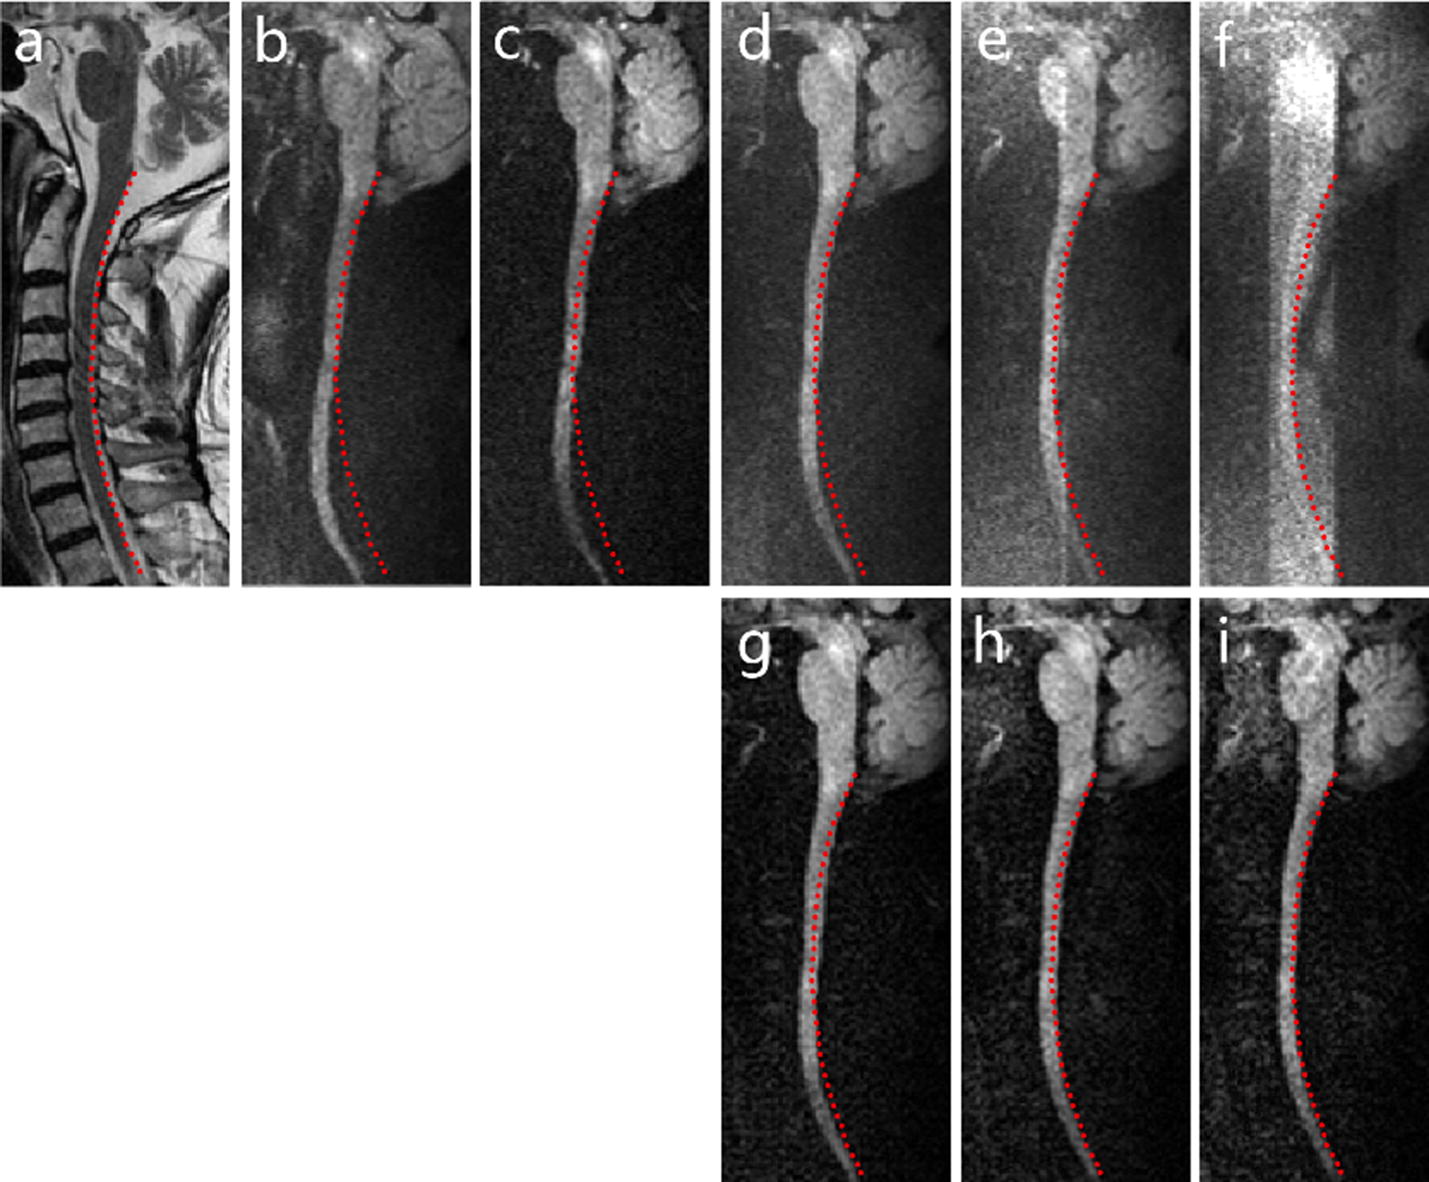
In vivo cervical spine images from one subject (male, aged 55). a The T2-weighted image shows slight cervical disc herniation. b The full FOV DWI with R = 2. c The fully sampled reduced FOV DWI. For R = 1.5/2/2.5, the SENSE magnitude average and ZOOM-SENSE results are shown in d–f and g–i respectively
